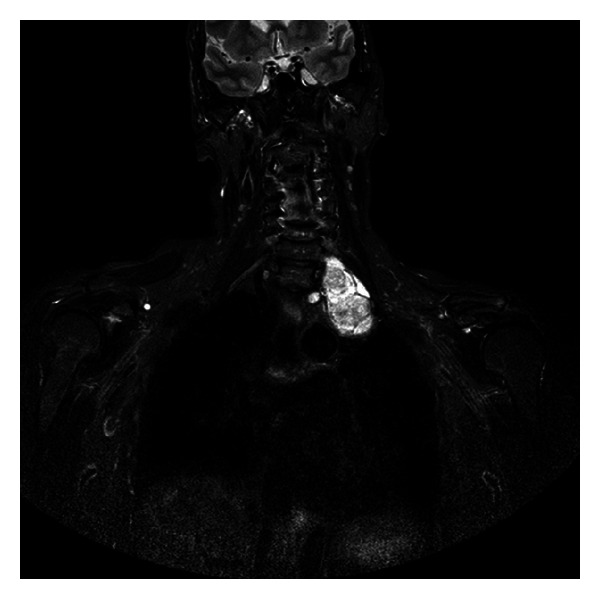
Magnetic resonance imaging (MRI) showing a left posterior mediastinal schwannoma extending to the cervical region through the thoracic inlet.
